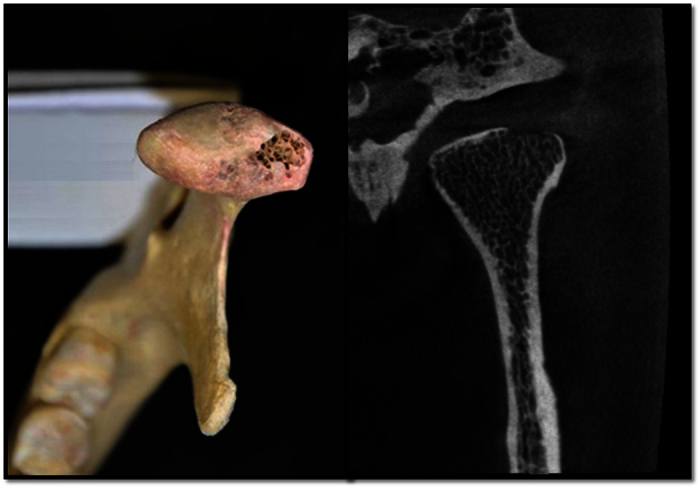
Human skull TMJs osseous defect sample and the corresponding CBCT images.
